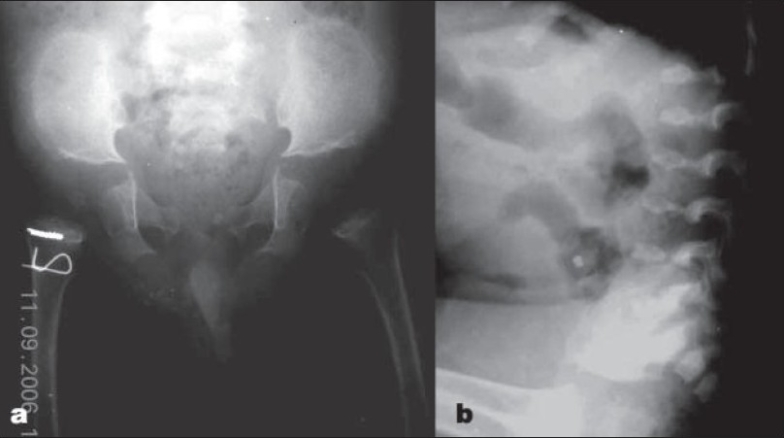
2 year old (younger) sibling. (a) Radiograph (anteroposterior view) of pelvis (in younger child) showing milder changes compared with that seen in elder sibling. (b) Radiograph (Lateral view) of Lumbosacral spine (in younger child) showing platyspondyly
